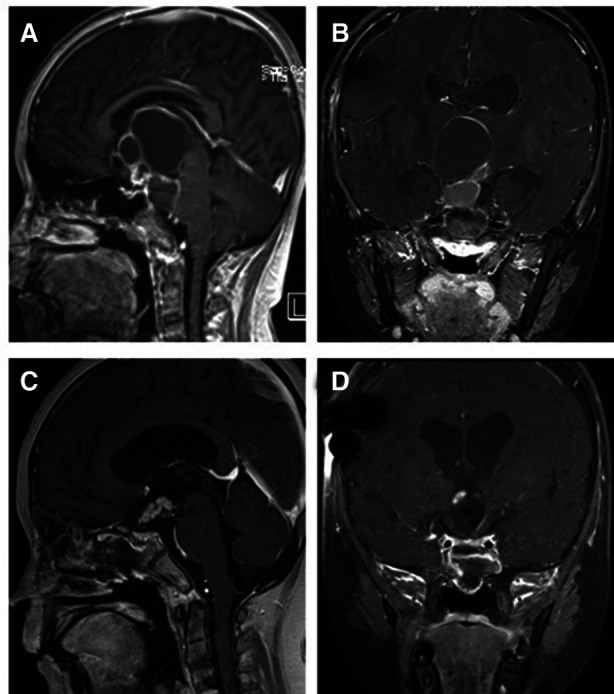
T1-weighted MRI image with contrast enhancement of suprasellar craniopharyngioma. (A) Preoperative sagittal view. (B) Preoperative coronal view. (C) Postoperative sagittal view. (D) Postoperative coronal view. Note in (C) and (D) the autologous fat employed intradurally to fill empty space within the sellar after tumor removal.
